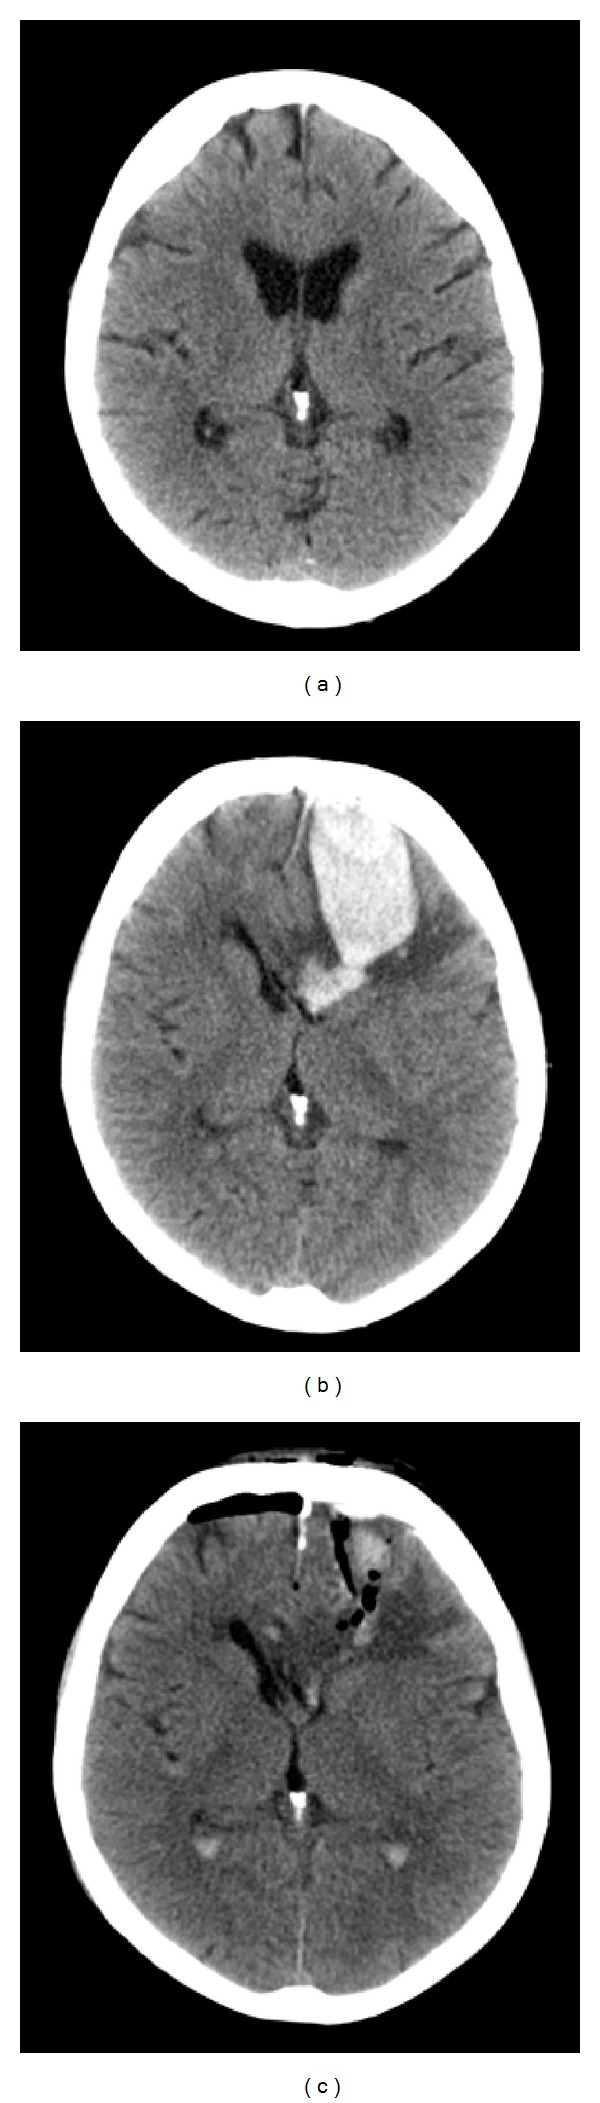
CT images performed initially after the head injury (a), after neurological deterioration (b), and after surgical haematoma evacuation (c).
